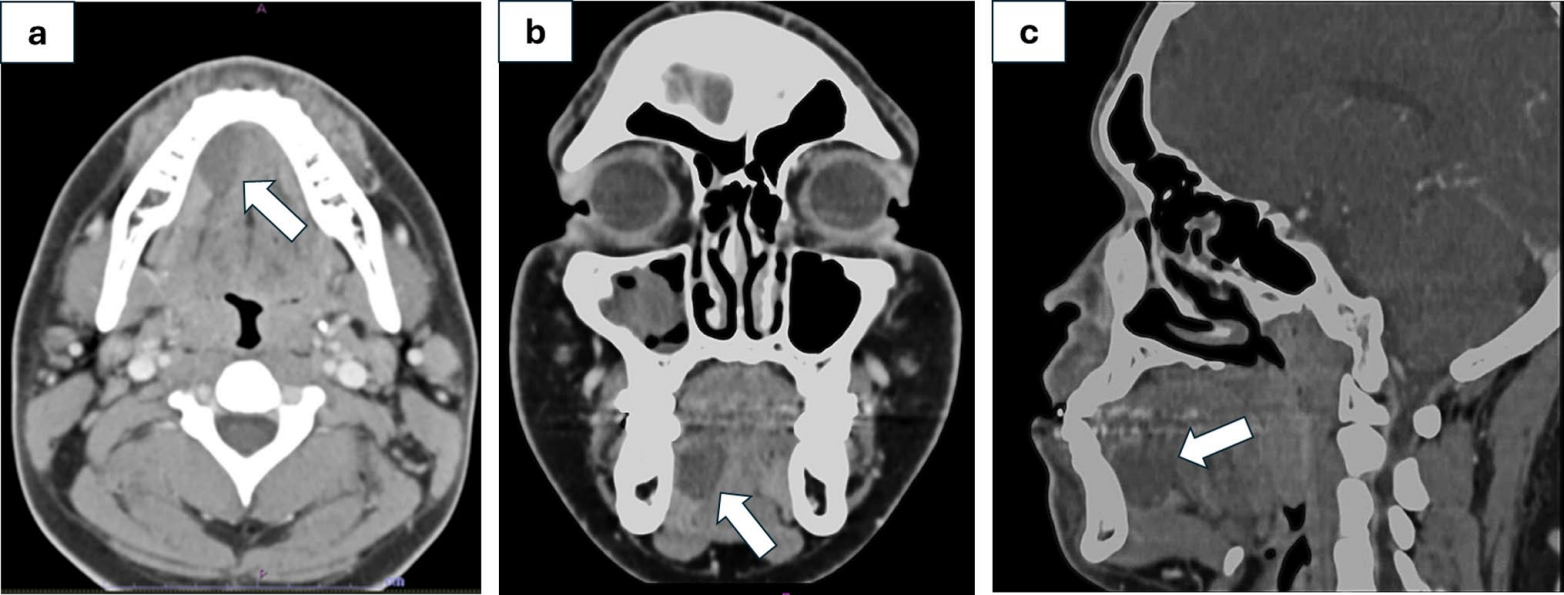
Computed tomography scan images show cystic mass appearing in the right anterior sublingual space (with arrow). a Coronal view. b Sagittal view. c Axial view
